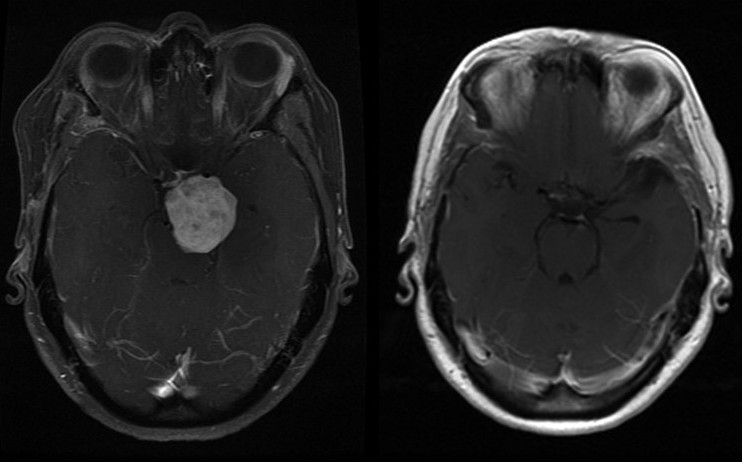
An axial gadolinium-enhanced contrasted T1-weighted MRI scan showing a large parasellar/ suprasellar mass with significant brainstem compression (a ). A postoperative axial gadolinium-enhanced T1-weighted MRI contrast scan 3 months later showing a complete resection of the mass with brainstem compression reversed (b)
